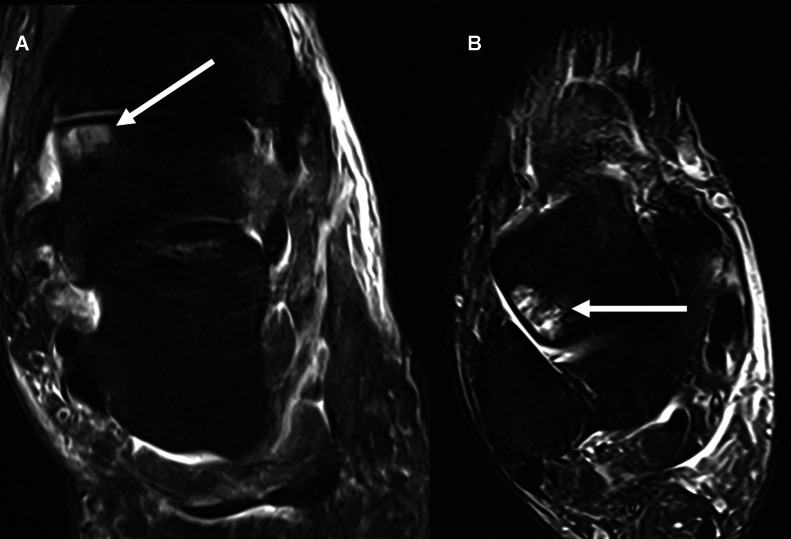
Postreduction coronal (A) and axial (B) proton density fat-saturated MRI sequences of the right ankle 2 weeks after the acute injury. There is a subtle impaction injury of the medial talar head articular surface with associated underlying marrow oedema like signal (white arrows).
